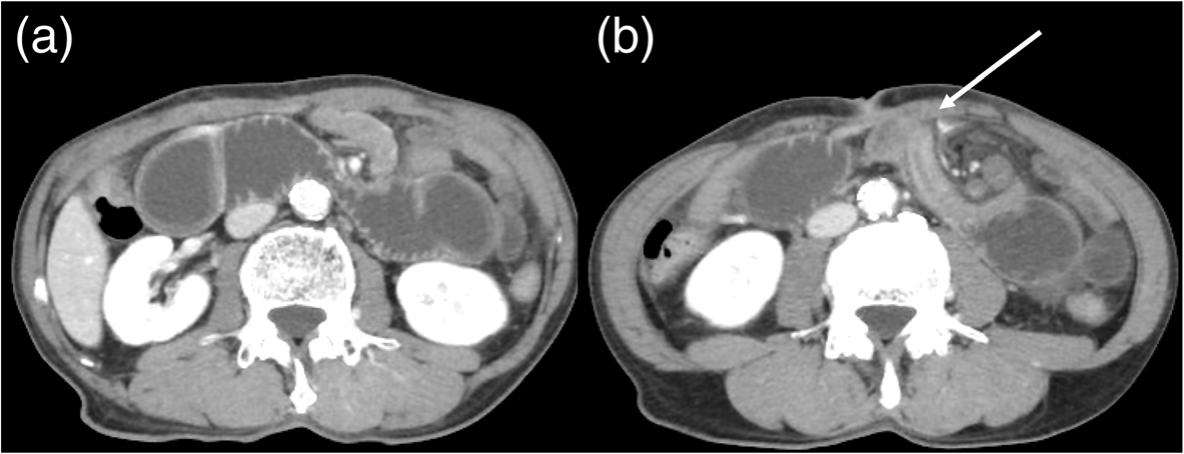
a. Computed tomography showing a dilation of the duodenum in a 62-years-old man who had been complaining of acute upper abdominal pain for 18 months after esophagectomy. b. An obstruction of the jejunum, at the site of the feeding jejunostomy, was identified (whirl sign; arrow), with twisting of the mesenteric vessels
